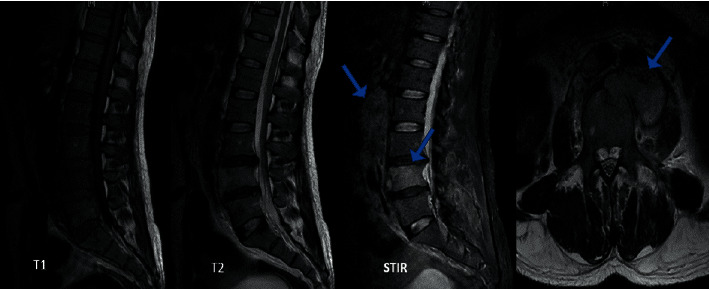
Lumbar spine and pelvis magnetic resonance imaging.
